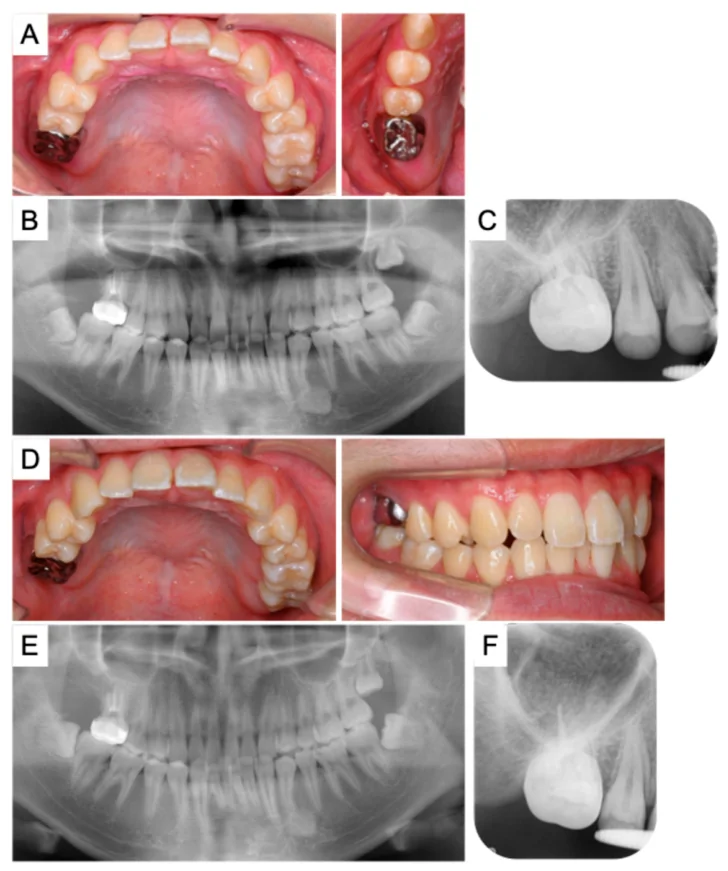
Intraoral and radiographic findings during follow-up. Follow-up was continued, and radiographic examinations were performed at 15 years and 5 months of age. These showed a radiolucency at the apex of the second molar, raising suspicion of apical periodontitis; however, the patient was placed under observation because no other pathological findings were present (A–C). At 16 years and 8 months of age, the radiolucency remained unchanged, and no recurrence of odontoma was observed (D–F). In addition, no changes were noted in the bone sclerosis of the mandibular left first premolar, and observation was continued. Oral management was maintained until 17 years 7 months of age, and no retreatment of the maxillary right second molar was required. At the time of this writing, the patient was continuing oral management in the Department of Special Care Dentistry.
